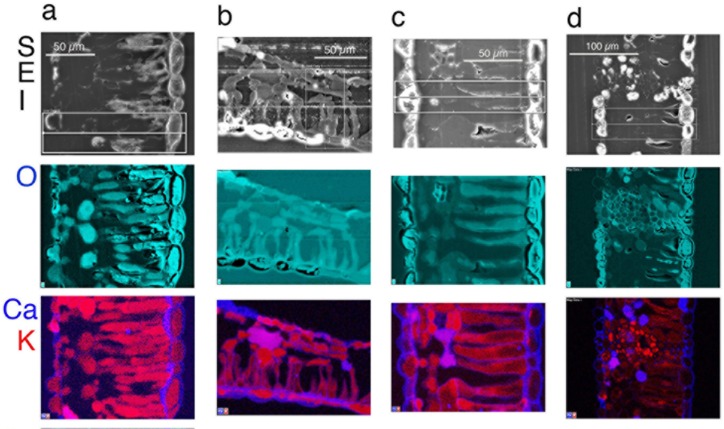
Secondary electron images (SEI) in the top horizontal panel, with corresponding colour-coded X-ray maps directly below, showing *in vivo* O and Ca-K maps in the two panels below. Leaf cross sections were taken from the following trees: (a) sugar maple uplsope (SM/U), tree no.1 in Table 1; (b) sugar maple downslope (SM/D), tree no. 3 in Table 1; c) red maple upslope (RM/U), tree no. 5 in Table 1; and d) red maple downslope (RM/D), tree no. 8 in Table 1. Upper epidermis at RHS in (a), (c) and (d), below in (b).
